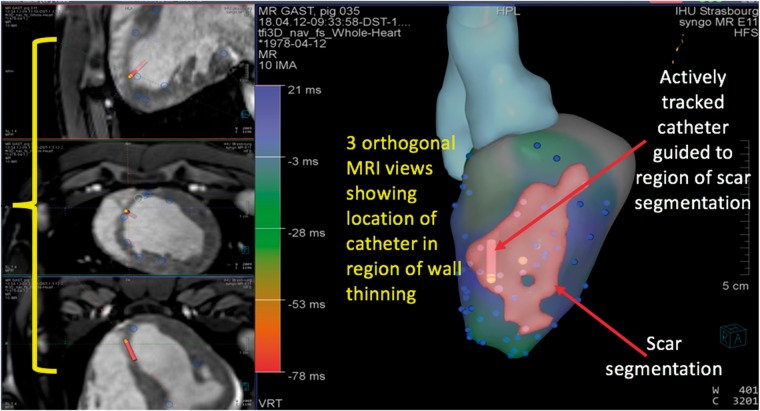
Representative depiction of image-guidance platform showing three orthogonal MRI views demonstrating location of catheter in relation to LV endocardium, three-dimensional segmentation of the left ventricle derived from MRI and scar segmentation from LGE images to guide EAM. EAM, electroanatomic mapping; LGE, late gadolinium enhancement; LV, left ventricular; MRI, magnetic resonance imaging.
